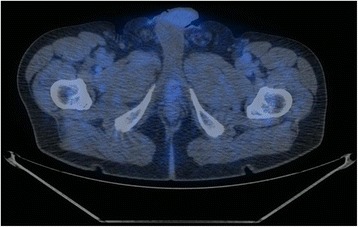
True positive case (PET/CT axial scan, PET only axial scan, MIP). This scan shows a focal uptake of 11C–choline localized in PB, right behind the bladder
